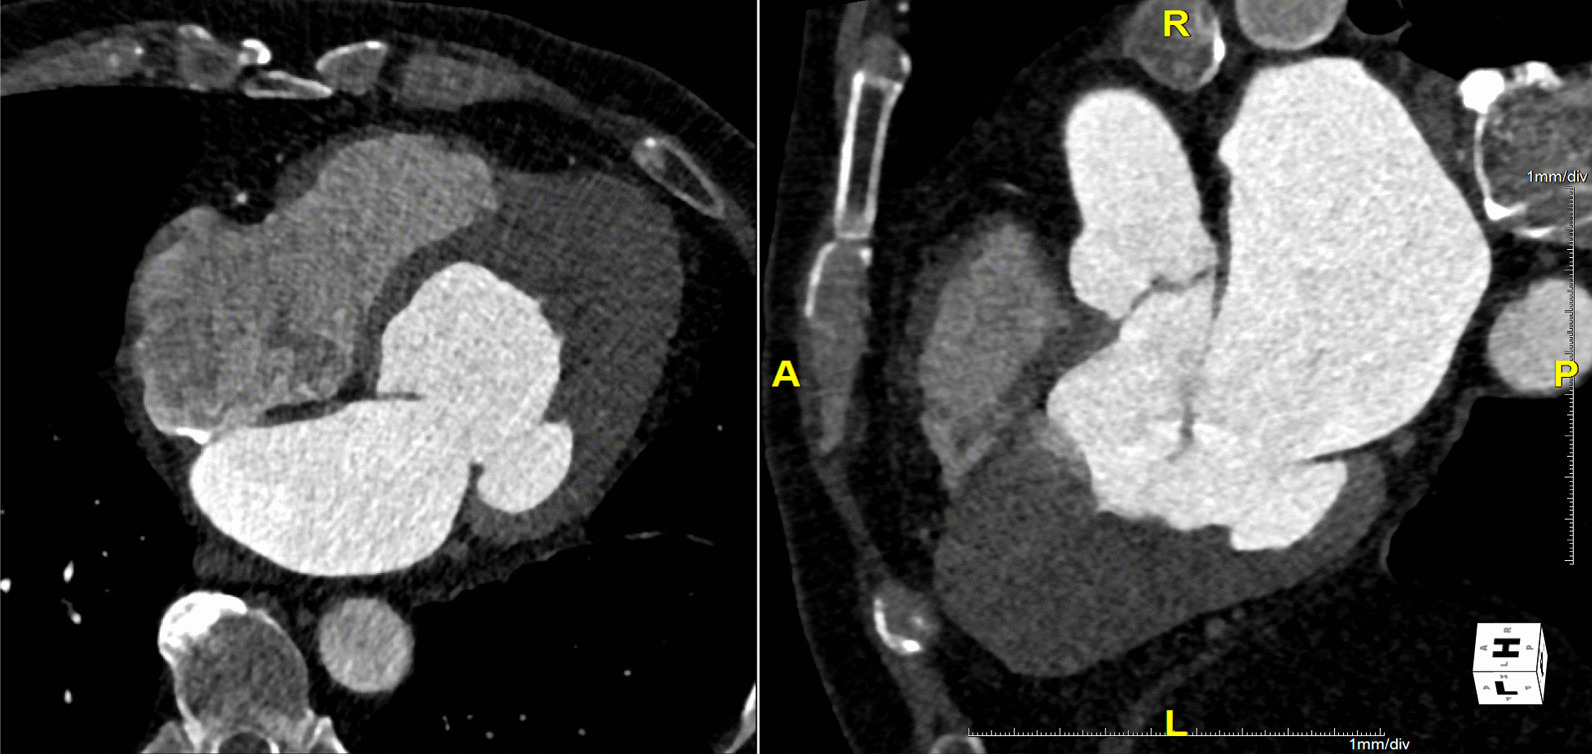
Preoperative CT images from Case 1. CT, computed tomography
